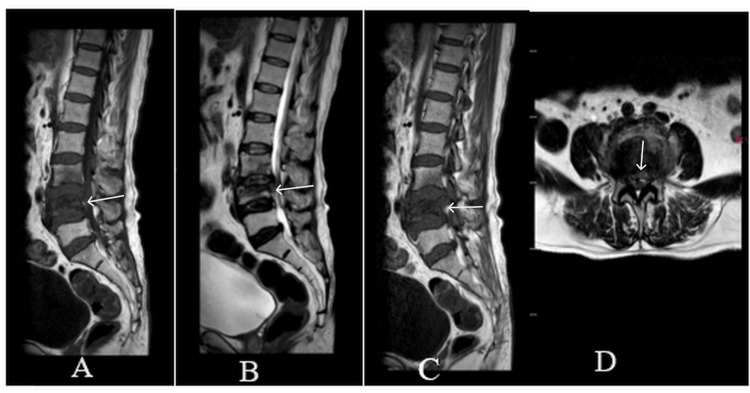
MRI of the lumbosacral spine (Case 6) (A) Sagittal T1-weighted image: hypointense marrow signal at L4 and L5 vertebral bodies (arrow). (B) Sagittal T2-weighted image: hyperintense inflammatory collections within the L4–L5 disc space (arrow). (C) Bone window image: adjacent vertebral destruction and endplate irregularity (arrow). (D) Axial image: epidural extension with mild canal compromise (arrow). Findings are consistent with infective spondylodiscitis at L4–L5.
